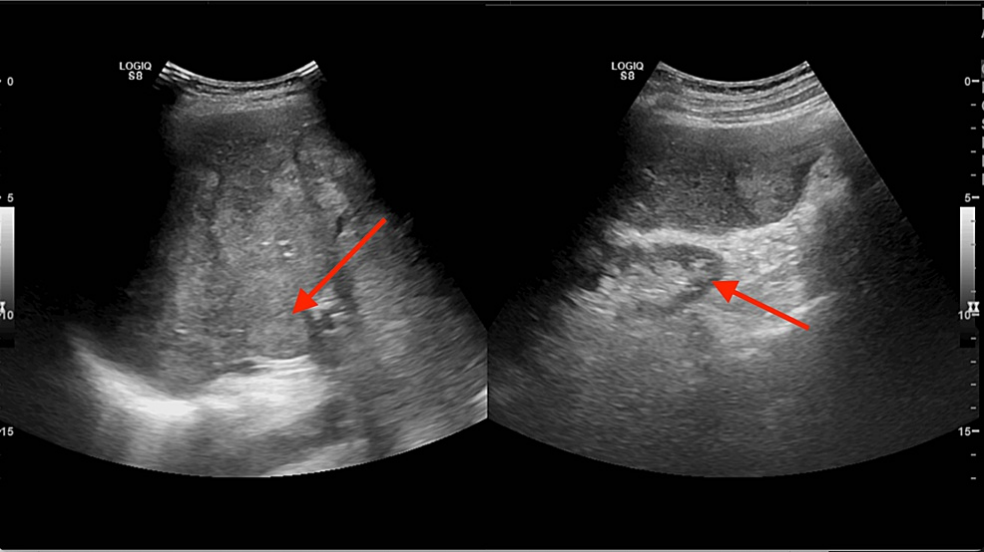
Abdominal ultrasound showing nodular lesions in the liver (red arrows)
